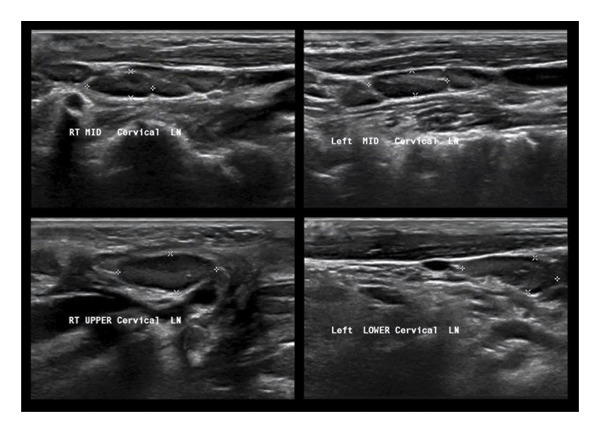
A follow‐up ultrasound imaging demonstrated normal findings with bilateral prominent cervical lymph nodes exhibiting preserved morphology, likely reactive to prior infection or inflammation, and no evidence of fluid collection.
